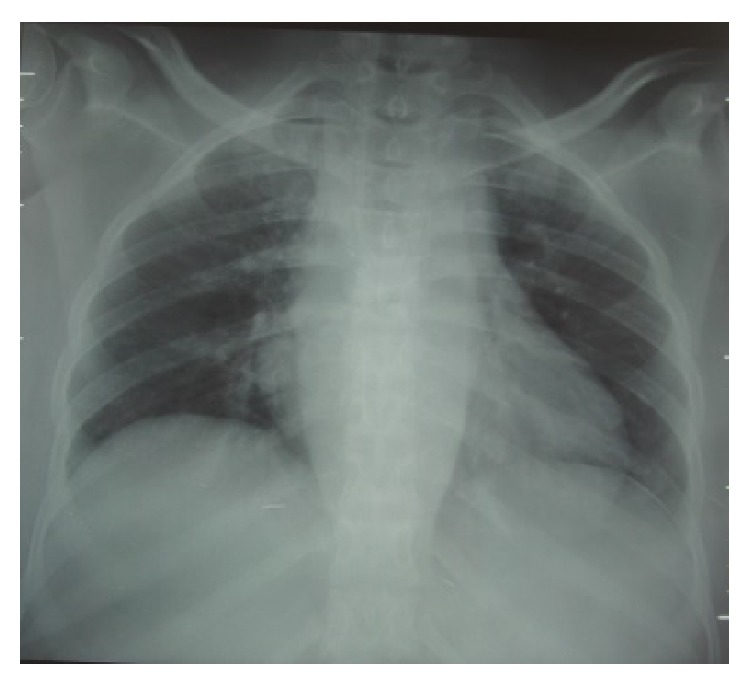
Chest X-ray showing enlargement of the mediastinum, consistent with tuberculous abscess mass.
